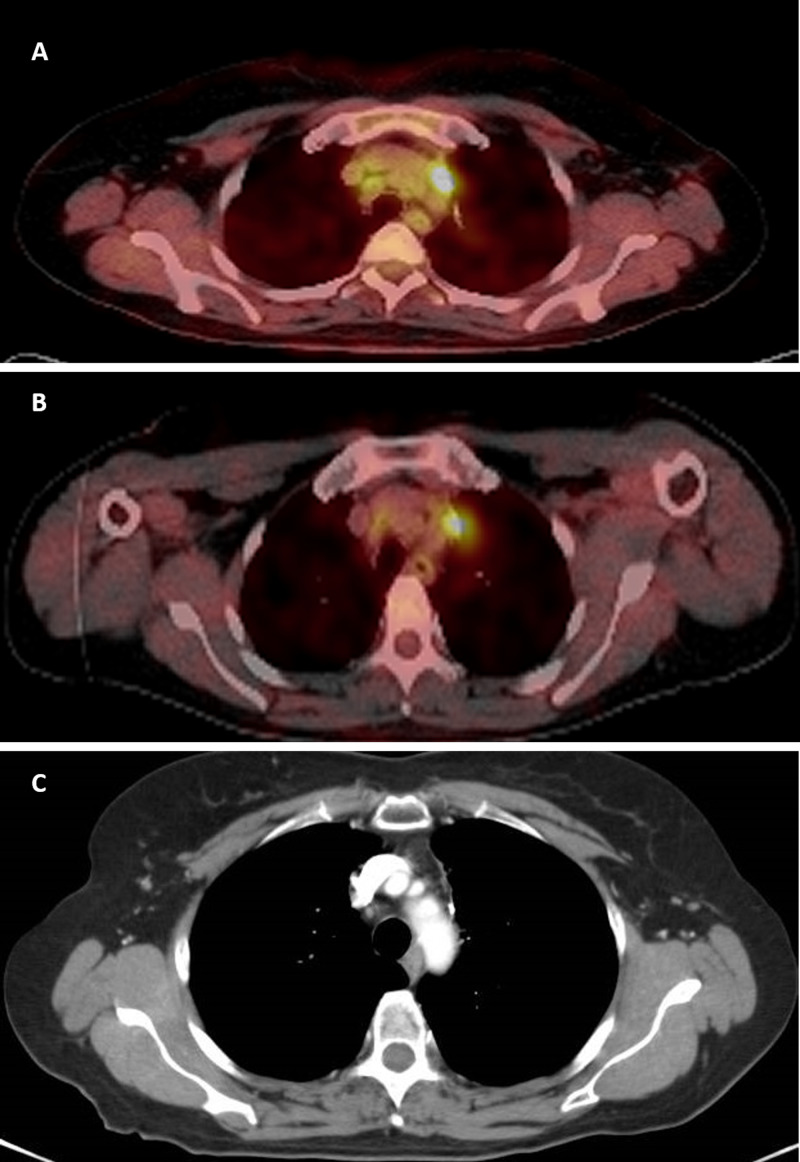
PET-CT A. PET-CT showing mediastinal hypermetabolic lymphadenopathies in the superior mediastinum, and paratracheal regions, SUVmax 6 (Date: Feb/2016). B. PET-CT showing mediastinal lymphadenopathies in superior mediastinum, paratracheal region and inferior left and right with decreased metabolism compared with previous study, SUVmax 2.99 (Date: Aug/2018). C. Chest CT showing absence of mediastinal metastases (complete response) (Date: Mar/2020). Abbreviations: CT, computed tomography; PET-CT, positron-emission computed tomography; SUVmax, maximum standardized uptake value.
